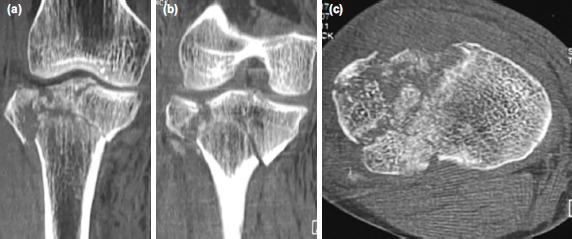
Case No.7, CT images of the knee showing comminution of the proximal tibia. (a) Coronal section image of anterior part of the tibial condyle. (b) Coronal section image of posterior part of the tibial condyle. (c) Cross-section of the tibial plateau revealing comminution.
